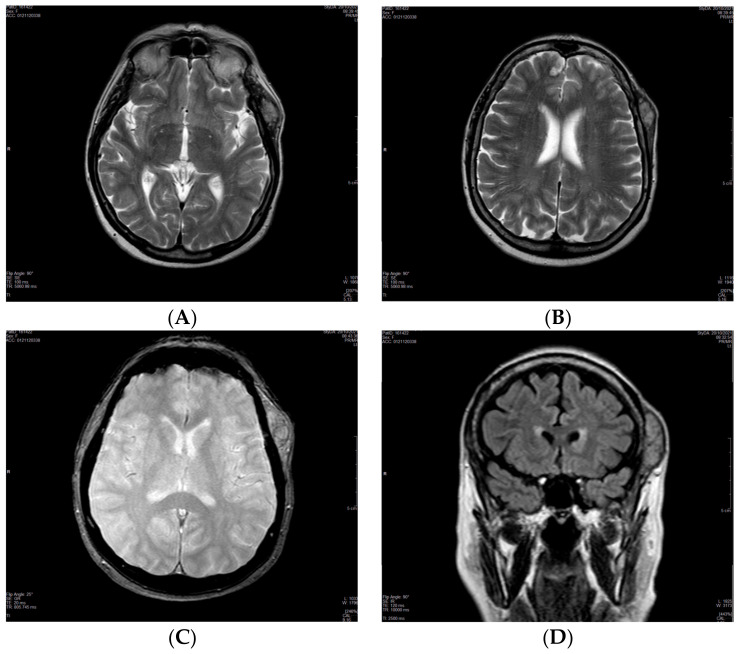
MRI in the axial (A–C) and coronal plane brain (D) shows a single 2.5 × 5 cm soft tissue temporal lesion in the left side with moderate contrast impregnation (C).
